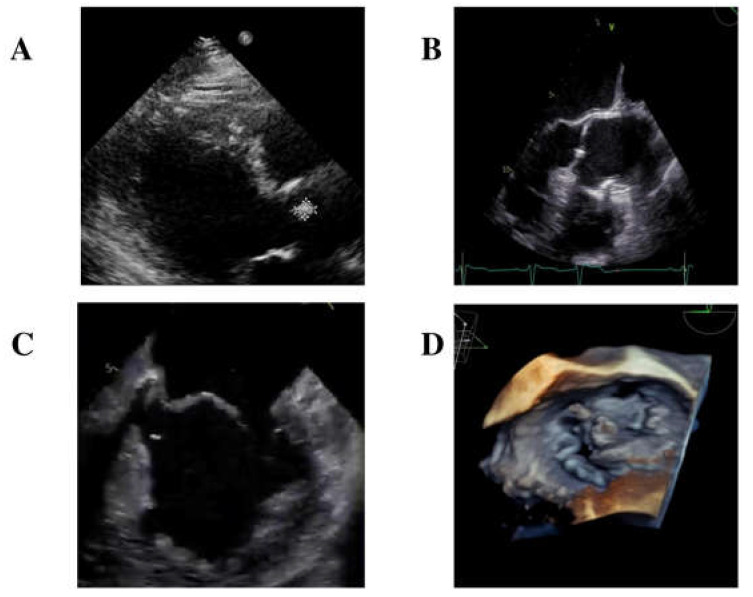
Transthoracic echocardiogram showing a mobile isoechoic formation at the right cuspid of the aortic valve (A). Transoesophageal echocardiogram image depicting a mobile isoechoic formation measuring 0.7 mm × 0.7 mm on the right cusp of the aortic valve (B); a mobile isoechoic formation on the posterior mitral leaflet with fragmentary flail of P2 due to rupture of the chordae tendineae (C); three-dimensional imaging of the mitral valve with the mass seen on P2 scallop (D).
